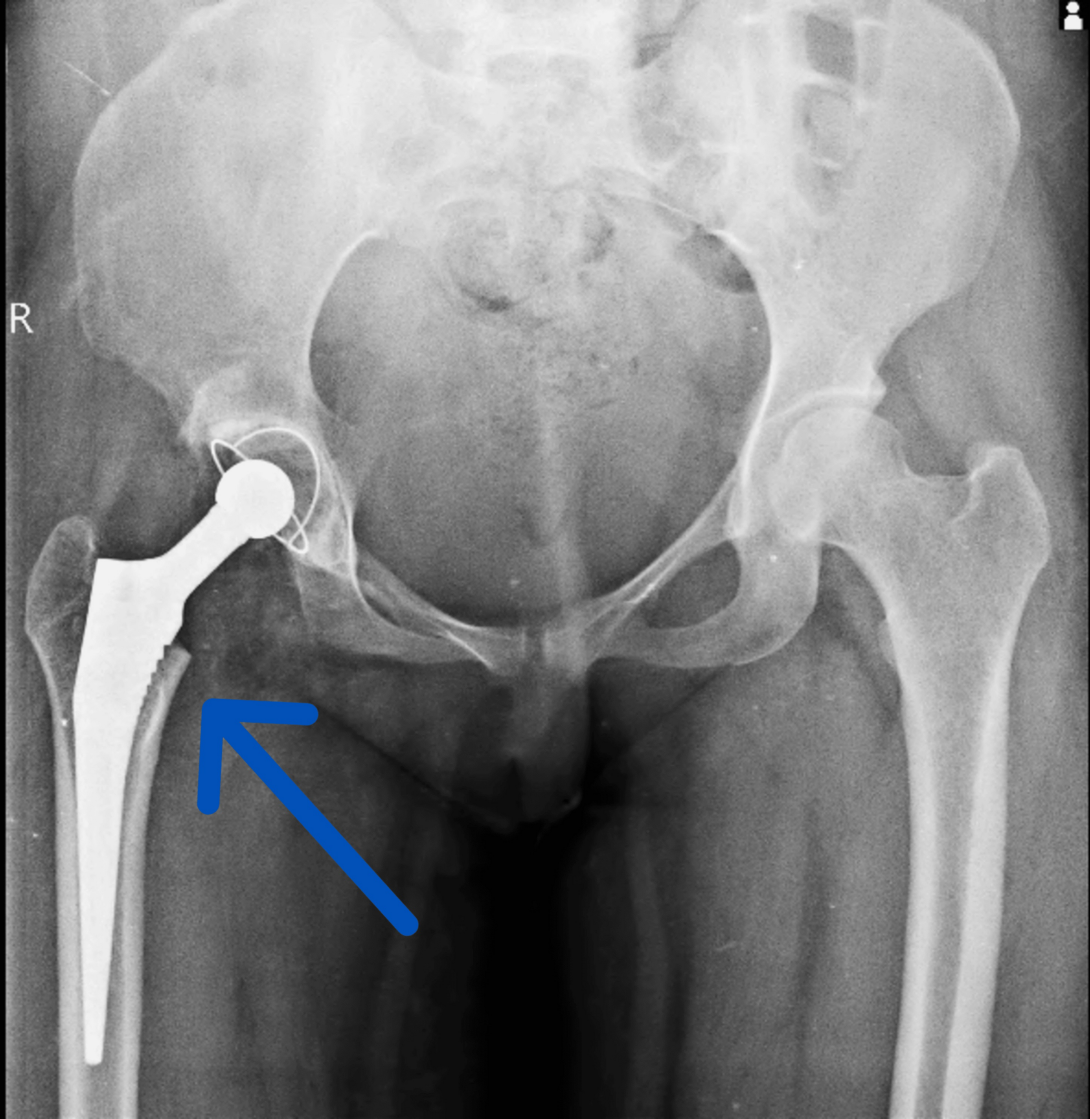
Postoperative X-ray The X-ray shows a cement-less total hip replacement prosthesis.
